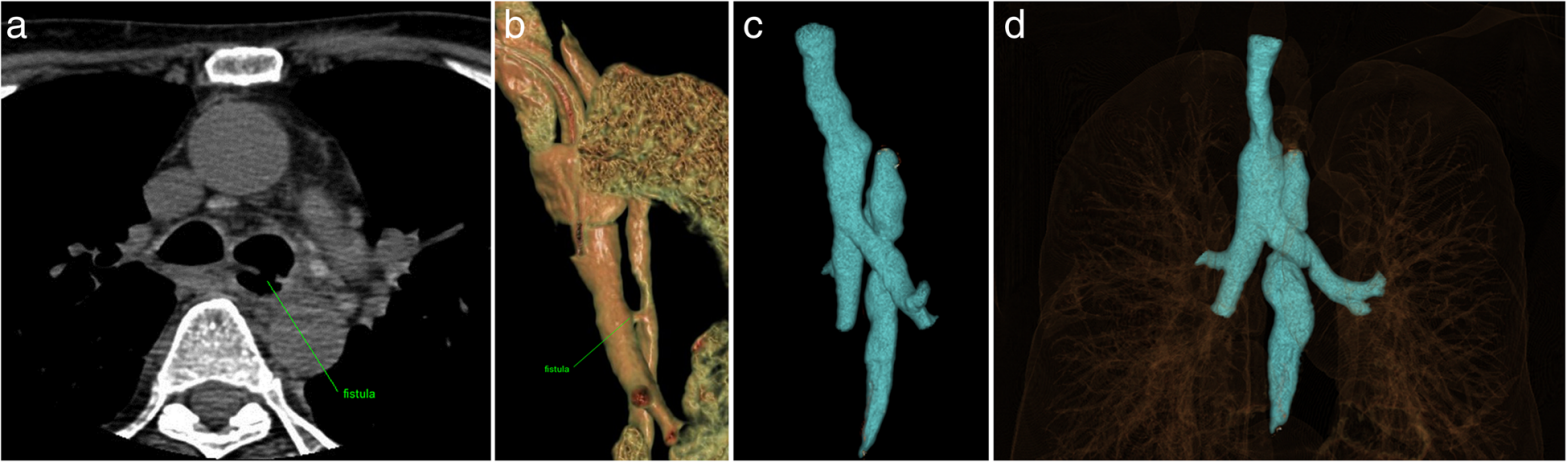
Bronchoesophageal fistula before primary resection and after esophageal bypass surgery. a Preoperative computed tomography revealing fistula between esophagus and left main bronchus, approximately 1 cm from tracheal bifurcation (green arrow). b Preoperative three-dimensional rendering of fistulous tract (green arrow). c, d Three-dimensional rendering 5 months after the esophageal bypass surgery revealing esophagus resected above and below the bronchoesophageal fistula level, leaving this section in situ on the posterior wall of the left main bronchus as a neo-pseudo-diverticulum. Substernaly interposed gastric conduit is not shown
